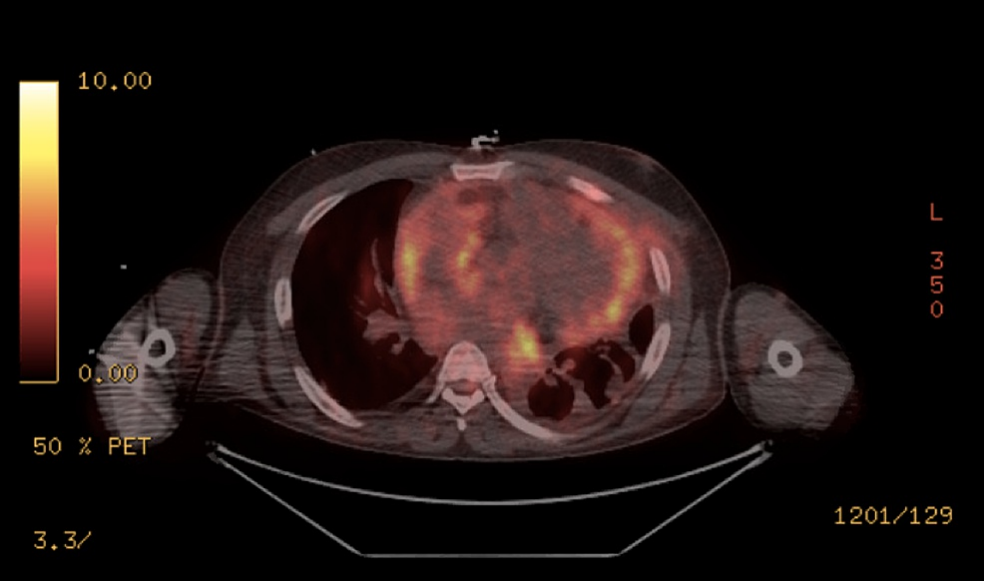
Axial view of PET showing increased FDG uptake in the pericardium PET, positron emission tomography; FDG, fluorodeoxyglucose
